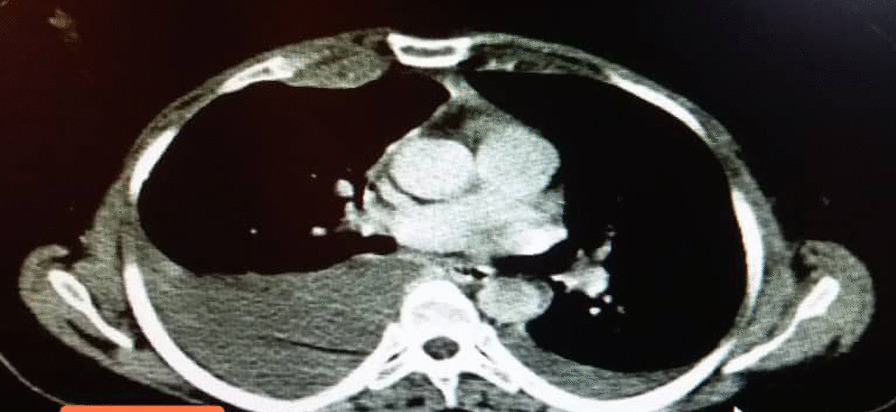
The computed tomography showed a severe right pleural effusion with right inferior lobe atelectasis
